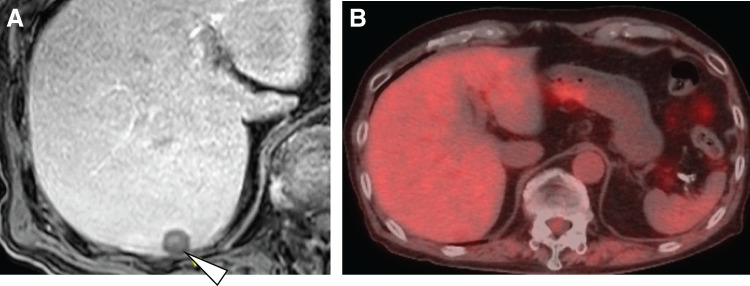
Preoperative imaging of the liver lesion. (A) Preoperative MRI imaging. (B) Preoperative PET-CT imaging. PET, positron emission tomography
